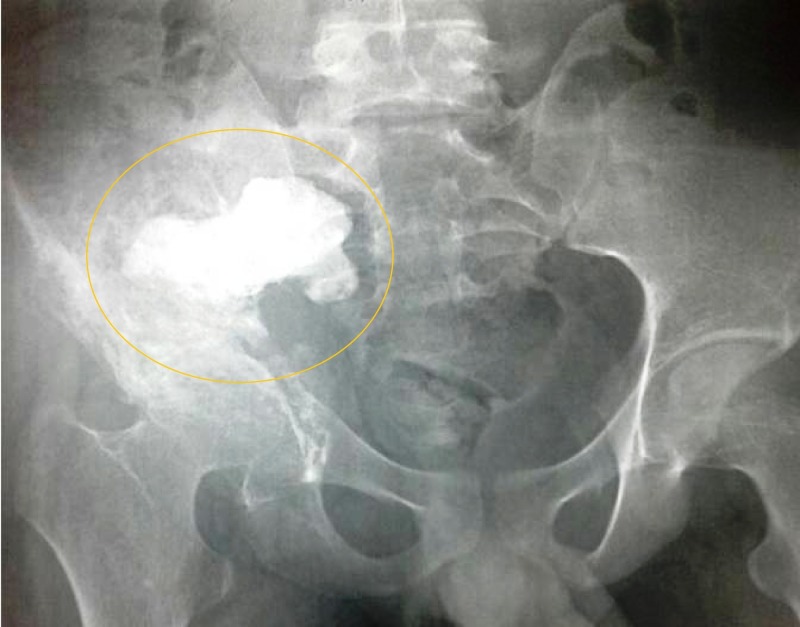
Post-procedure pelvic radiograph showing bone cement in place in the right ilium and sacroiliac joint (anteroposterior view; circle)
